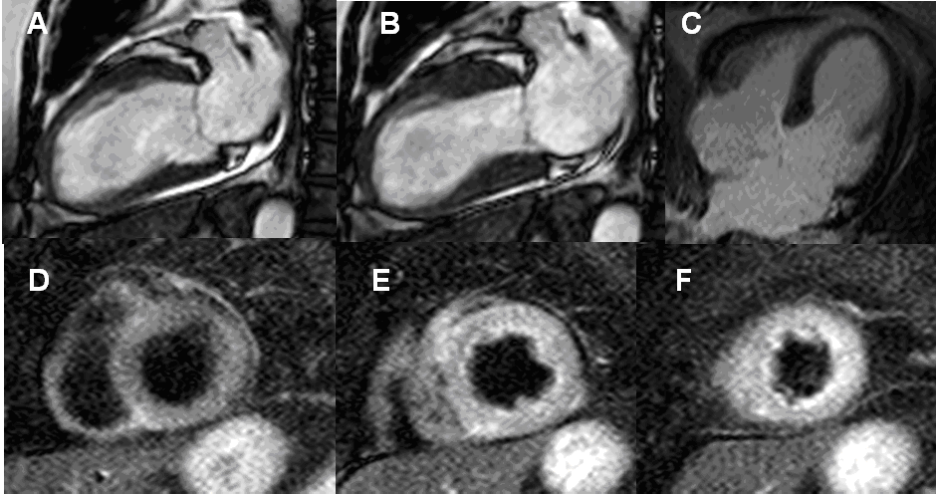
CMR Findings in a Patient With Stress-Induced Cardiomyopathy Takotsubo. There is characteristic apical contractile dysfunction (A, B) in the absence of late gadolinium enhancement (C).  T2-weighted images showing normal signal intensity of the basal myocardium (D), but global edema in the apical and midventricular myocardium matching the distribution of the LV wall motion abnormalities (E, F).
